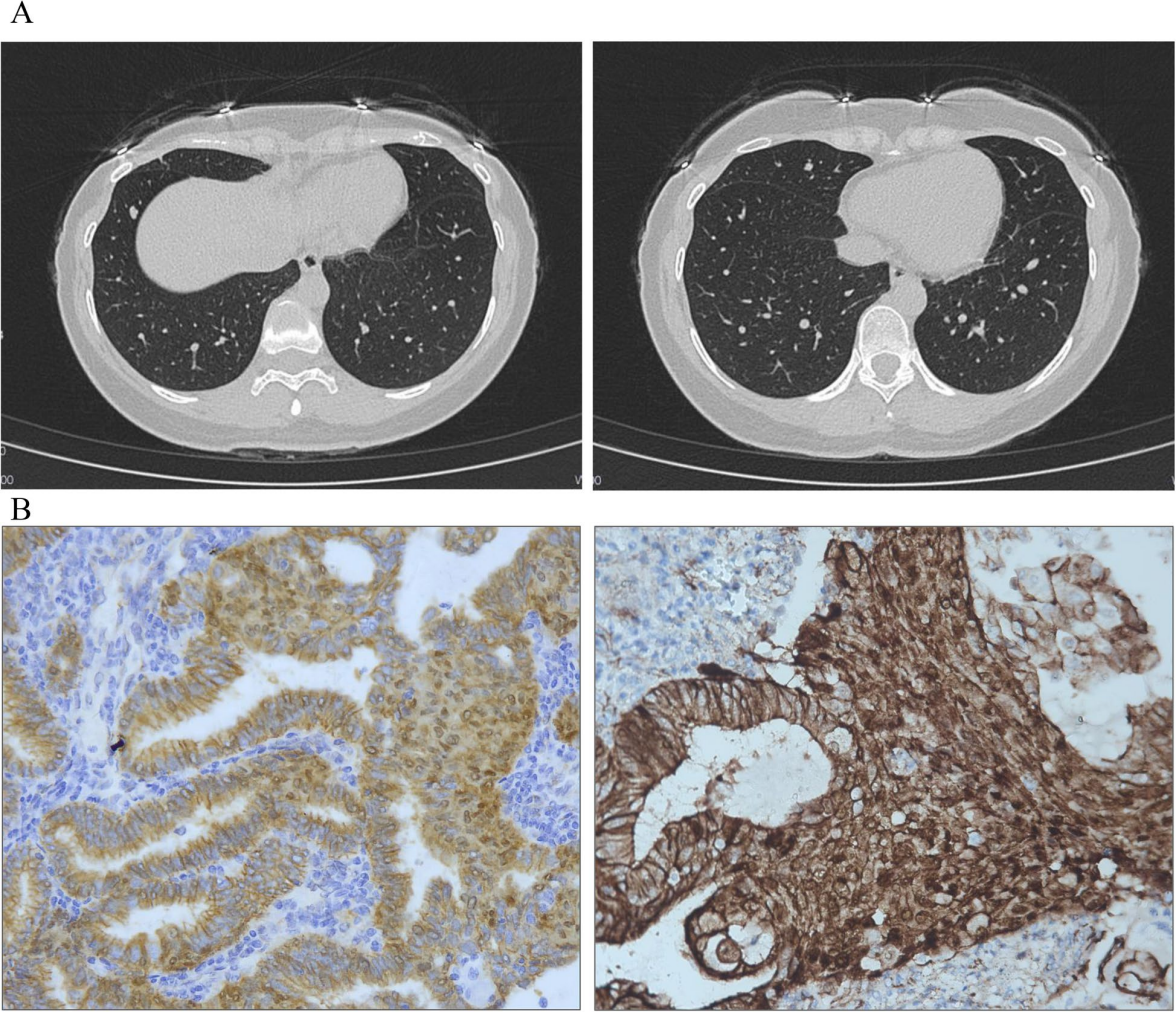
CT findings. A: CT scan of the chest showed lung metastasis. Left: Cross-section of a high-density pulmonary nodule in the anterior basal region of the right lower lobe. Right: Cross-section of a high-density pulmonary nodule in the right middle lobe of the lung. B: β-catenin was evaluated by IHC in primary and metastatic tumors. Clustered nuclear location of β-catenin in primary tumor (a) and lung metastatic tumor (b) (400x)
